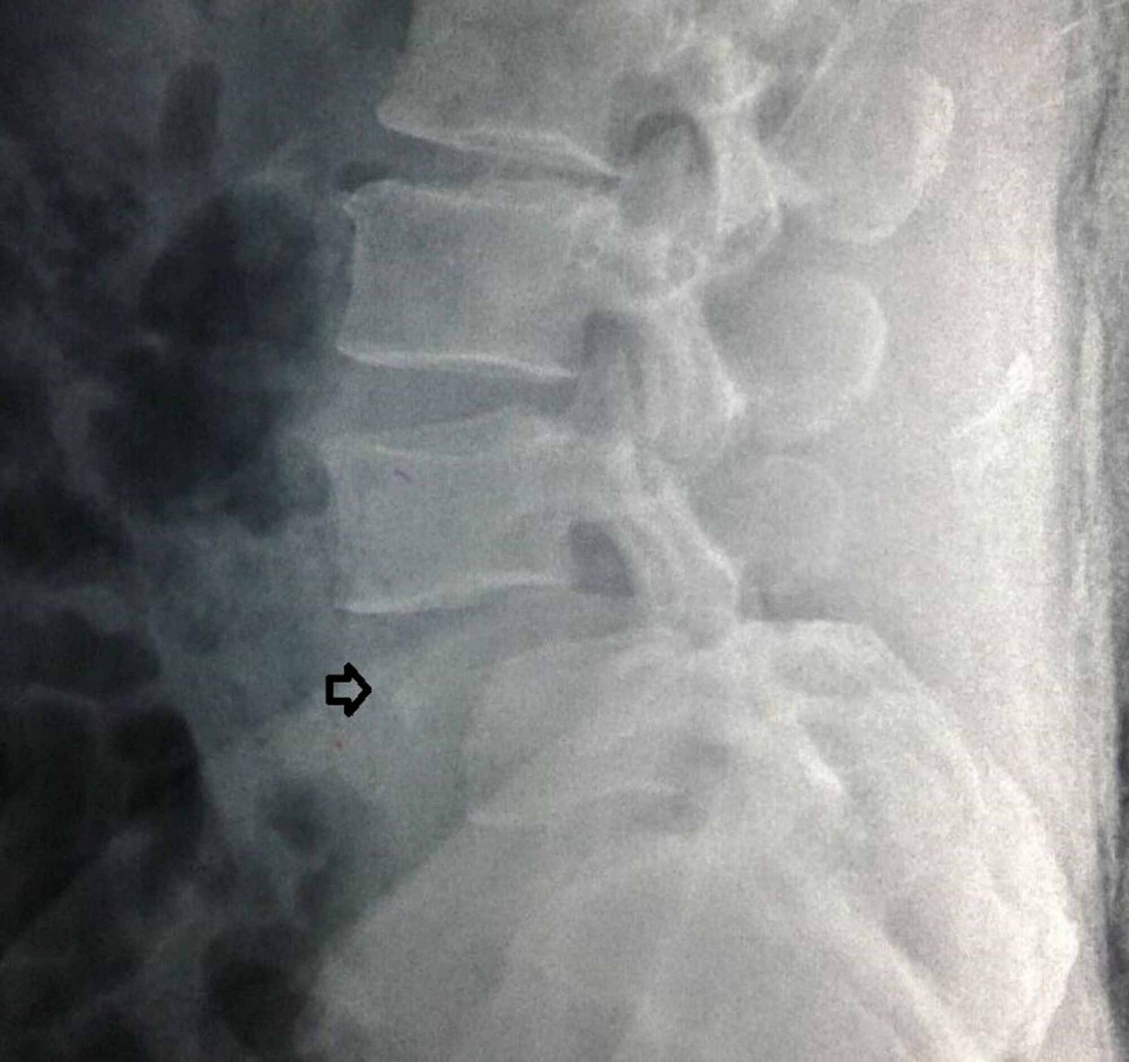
Preoperative supine lateral roentgenogram on admission of the lumbar spine showing Meyerding III anterolisthesis of L4. The black arrow shows the avulsion fracture of the anterior–superior vertebral corner.
